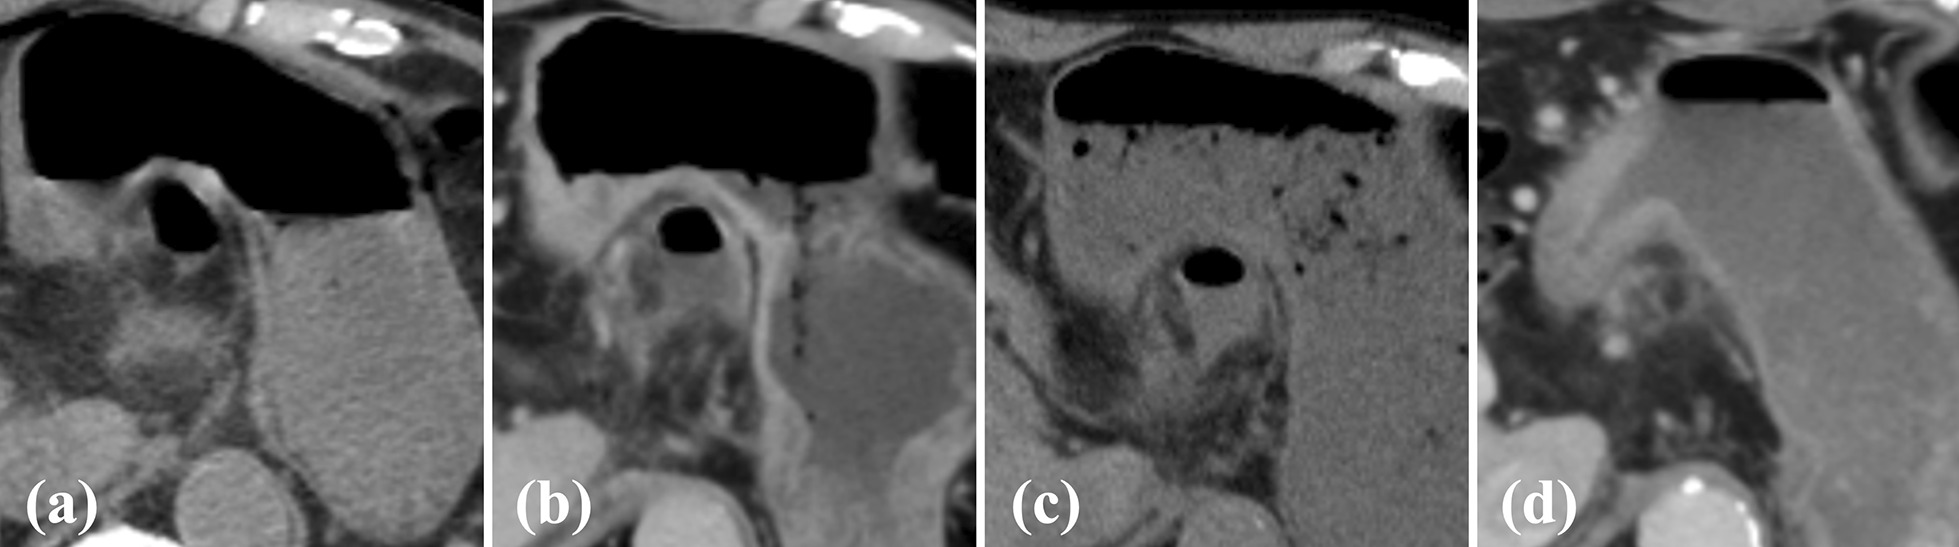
a On postoperative day 2, a computed tomography examination revealed the small amount of fluid collection abutting the dorsal area of the stomach. b On postoperative day 5, a follow-up computed tomography examination revealed an encapsuled and localized perigastric abscess. c, d The perigastric abscess had regressed on postoperative day 7 and had almost disappeared on postoperative day 34
